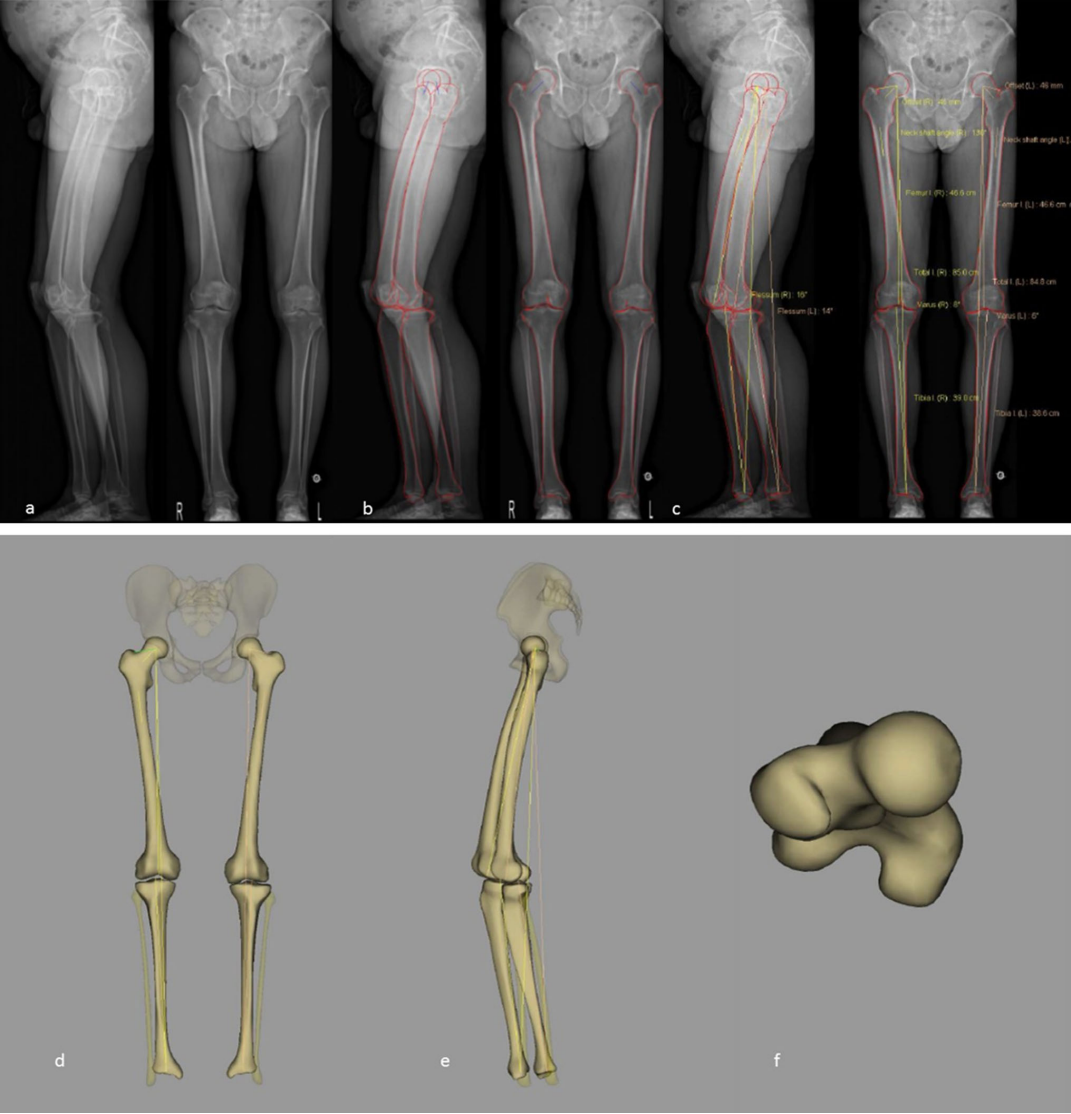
Example of a biplanar radiograph (a), with subsequent bony contour determination (b), measurements (c), and 3D reconstruction (d–e)
